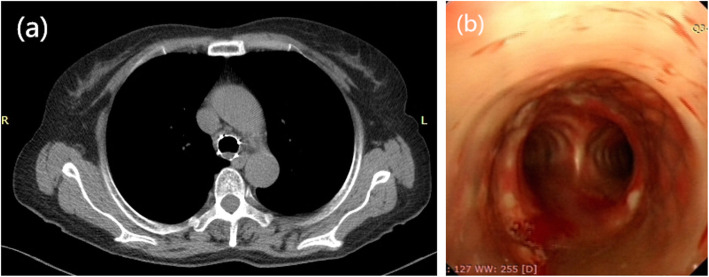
Chest CT (a) and bronchoscopy (b) before tracheal stent removal
